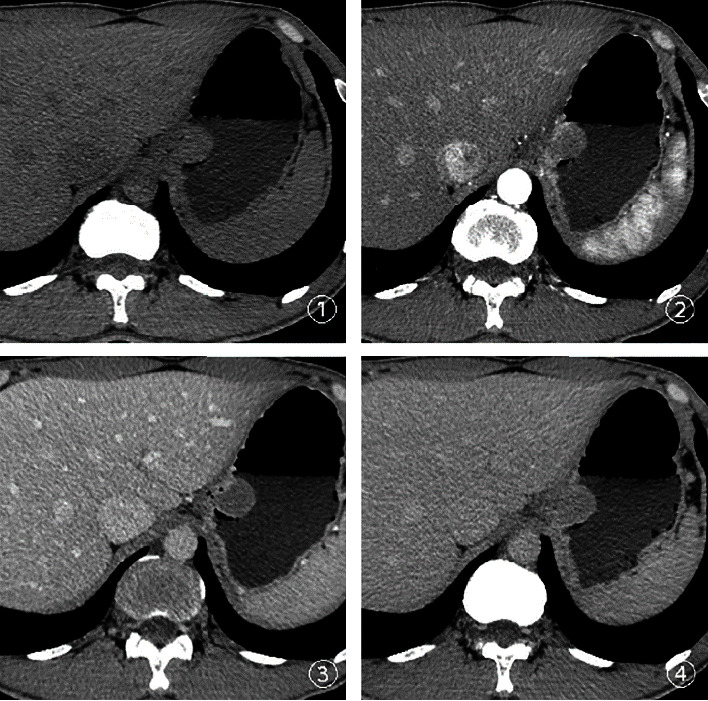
A 37-year-old man with small GLMs in the gastric wall. ① Plain CT scan showing a soft tissue mass growing in an oval cavity from the cardia, smooth with clear edge and a maximum diameter of 2.7 cm. The CT value was 38 Hu and the mass grew inside the cavity. ②–④ Enhancement scanning of the arterial phase, venous phase, and delayed phase, respectively, resulting in homogeneous enhancement of the mass.
